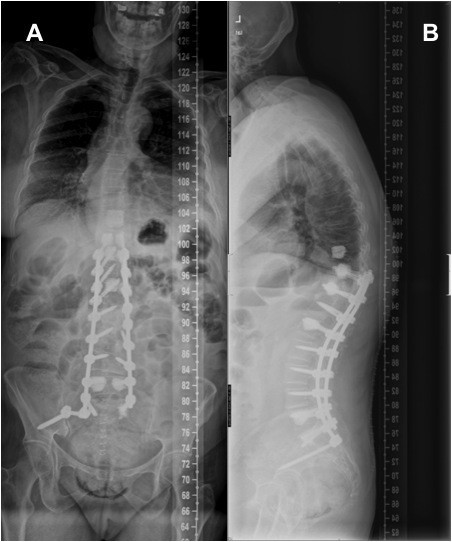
Postoperative p-a and lateral x-rays showing a posterolateral arthrodesis from T10 to S1 and iliac screw in the right side, augmented with cement at T10, T11, L1, L5 and S1. Prophylactic vertebroplasty at T9 was performed to avoid the “topping-off syndrome”. Balanced profile in both frontal and sagittal planes were obtained.
